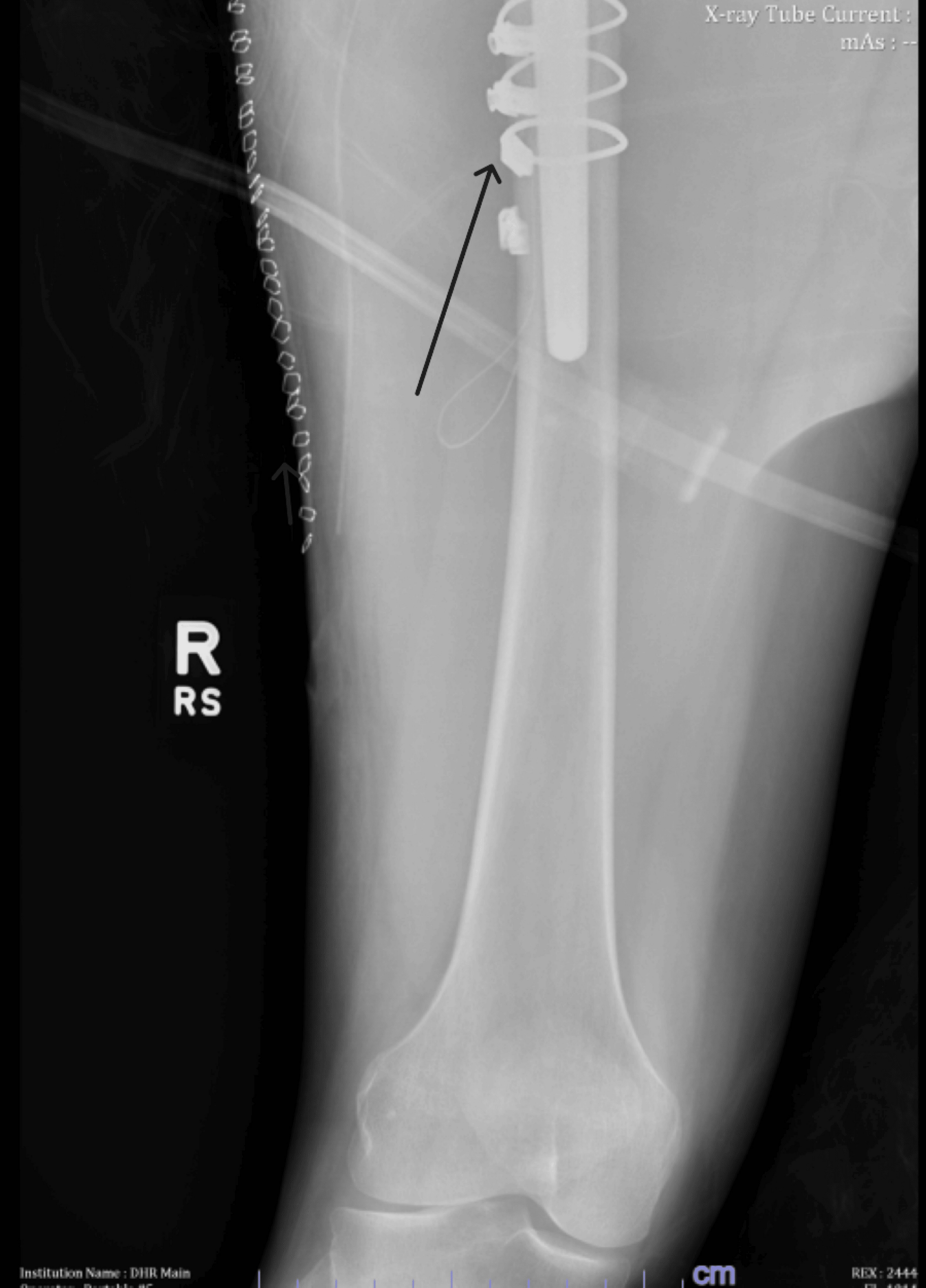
Postoperative AP X-ray of the right leg demonstrating the distal portion of the long stem femoral prosthesis Arrow indicates the cerclage wire AP, anteroposterior
